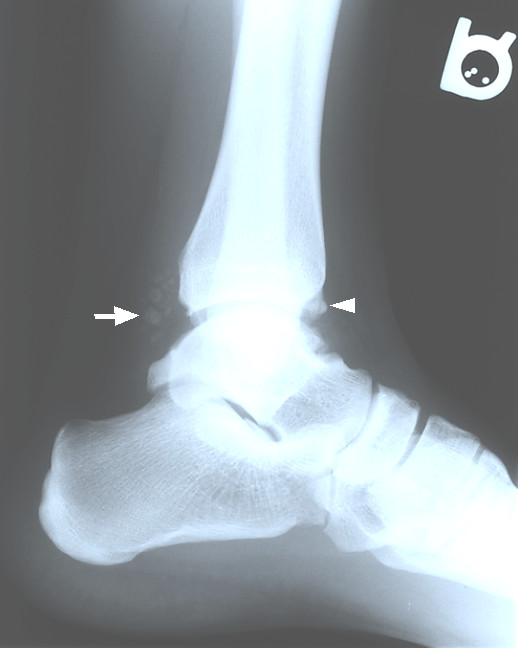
Lateral right ankle radiograph with evidence of calcified loose bodies (arrow) posterior to the talotibial joint. Small loose bodies are also seen anteriorly to the joint (arrow head).
